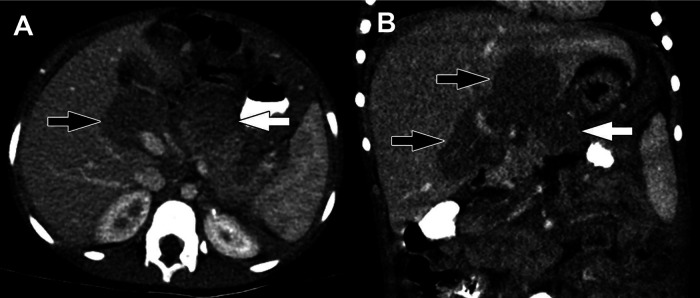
Burkitt lymphoma. (A) Axial and (B) Coronal reformatted images from a CT of the abdomen obtained in the portal venous phase following intravenous contrast material administration show hypoenhancing masses in the pancreas (white arrows) and porta hepatis (black arrows). Multiorgan involvement and infiltrative growth are highly suggestive of Burkitt lymphoma.
